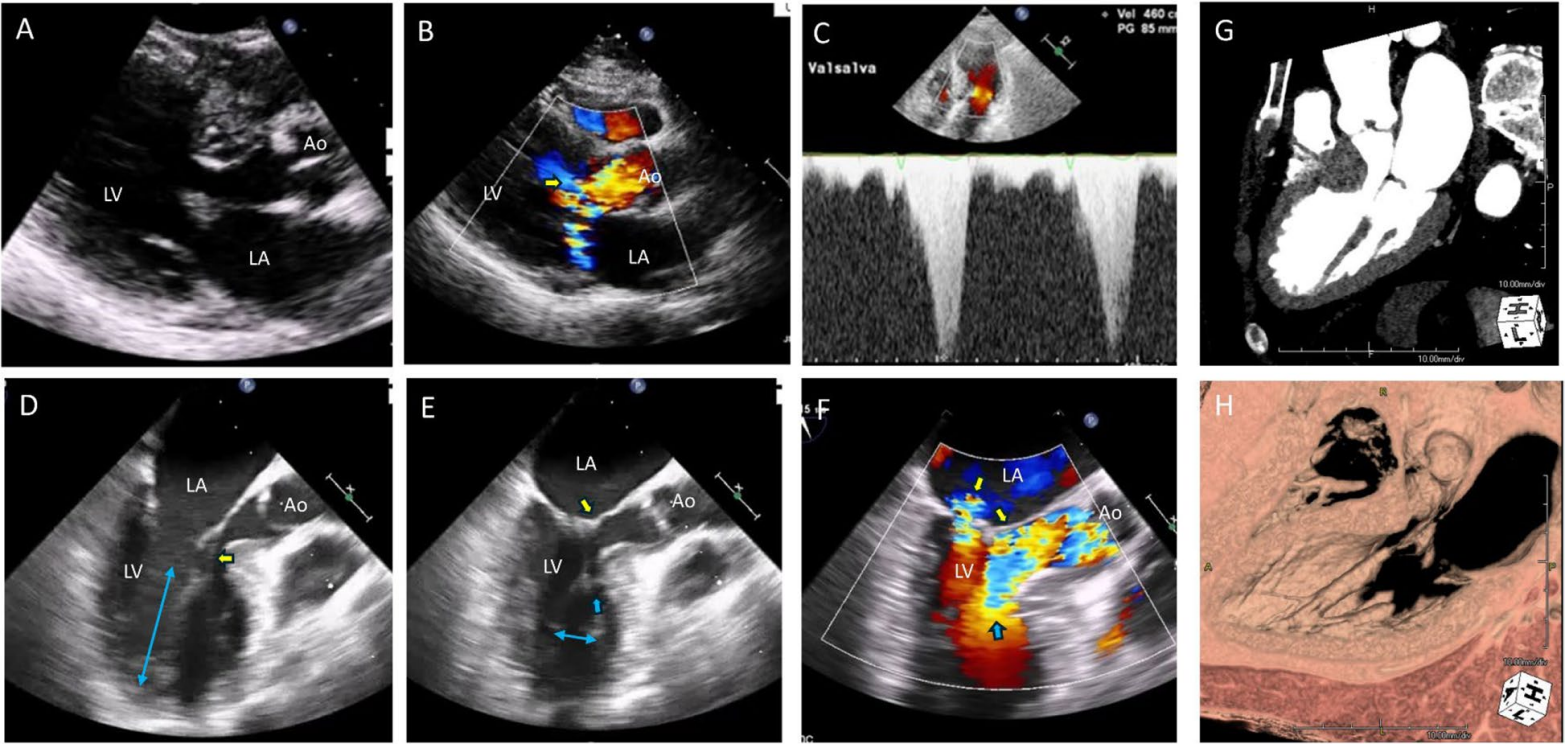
Representative echocardiographic and computed tomographic images showing an elongated papillary muscle causing significant left ventricular outflow tract obstruction without systolic anterior motion of the mitral valve. A, B Parasternal long-axis views with (C) Doppler tracing suggest typical left ventricular outflow tract obstruction. (D, E) However, transesophageal echocardiographic images clearly show that systolic anterior motion of the mitral valve is absent (yellow arrows): the thick and elongated papillary muscle (D, blue arrows) inserts into the mitral leaflet with thin chordae tendineae extension (D, yellow arrows), which almost contacts the hypertrophied septum during systole, resulting in outflow tract obstruction (E, blue arrow). G, H Computed tomographic images also demonstrate a thick and elongated papillary muscle inserting to the mitral leaflet with a thin chordae tendineae connection
